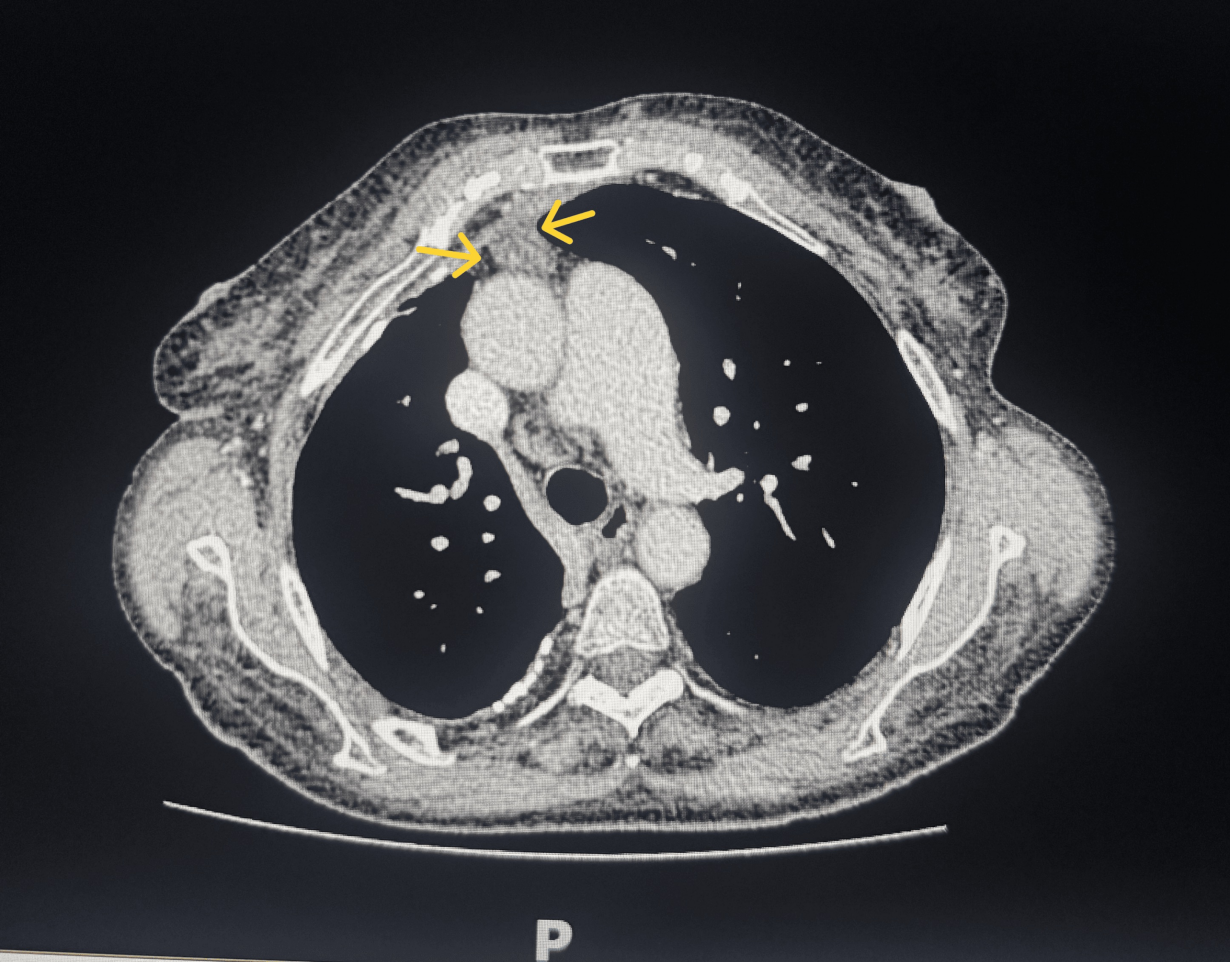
Chest CT image of the patient CT: computed tomography
